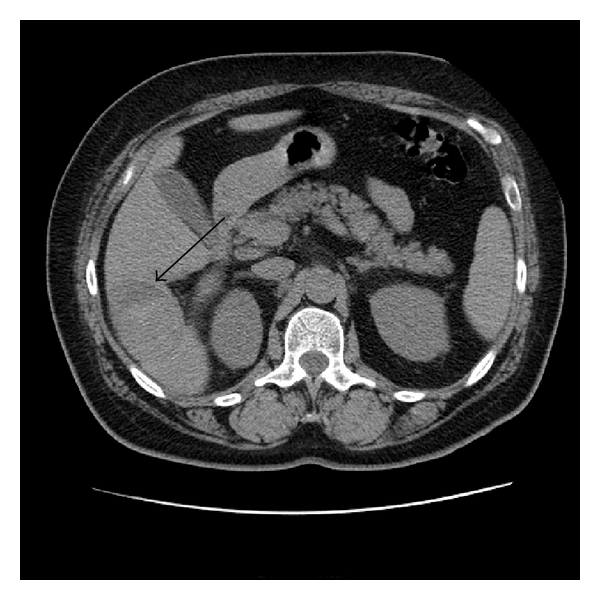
Unenhanced computed tomography of the liver demonstrates a 53.5 mm heterogeneous area in segment six (arrow).
